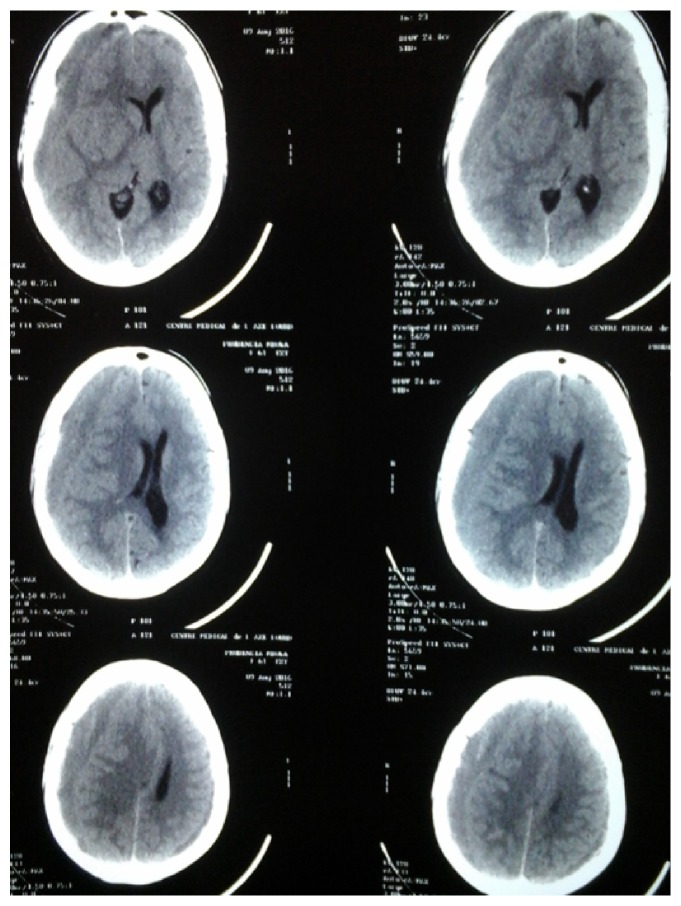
Computed tomography scan of the brain showing a right chronic subdural hematoma with midline shift.
